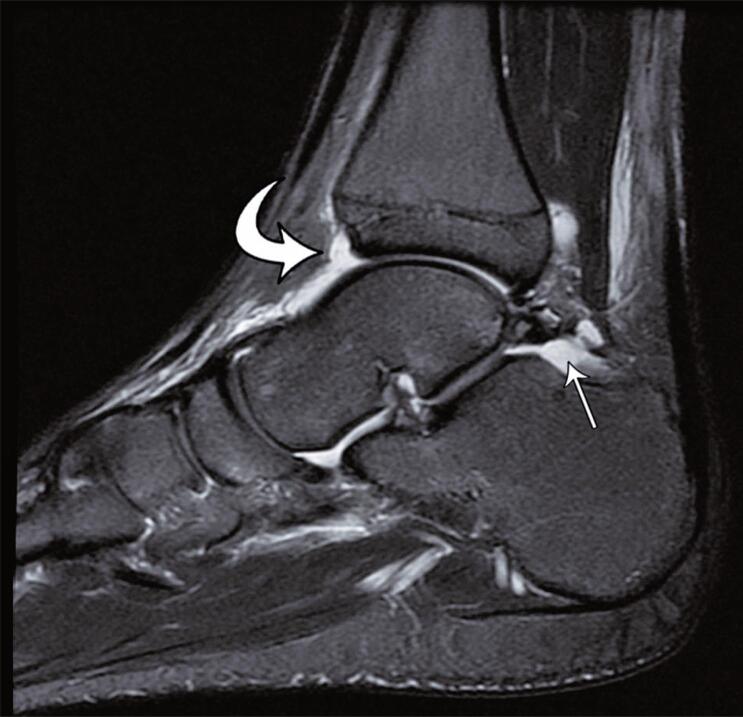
Sagital T2-weighted fat-suppressed magnetic resonance image demonstrating moderate effusion in tibiotalar (curved arrow) and subtalar joints (thin arrow)
